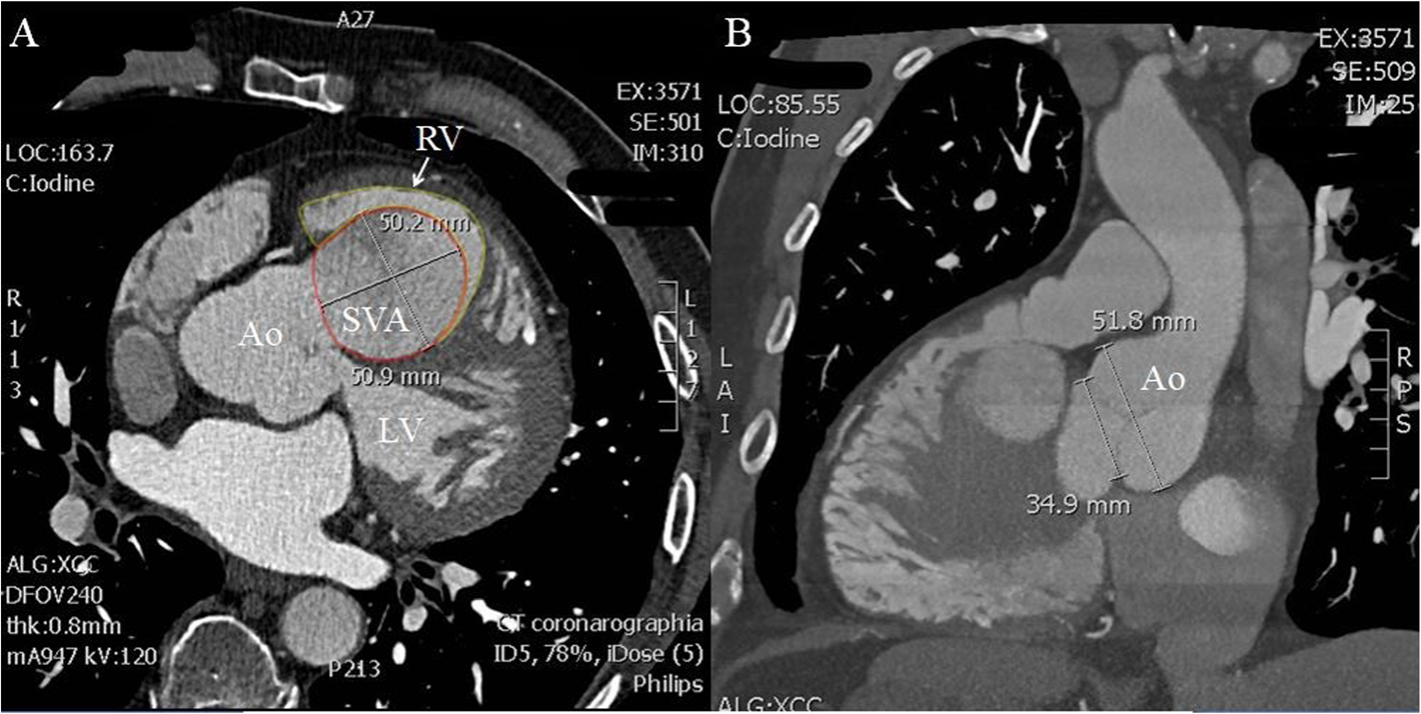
Computed tomography angiography. a – Transverse section showing the origin of the SVA from the right aortic cusp, occupying most of the right ventricle (yellow line) which is visible in this incidence as a thin zone around the aneurysm (red line). b – Sagittal section showing the diameters of the aortic valve and aortic root at the level of the sinuses of Valsalva. SVA = sinus of Valsalva aneurysm; Ao = aorta; LV = left ventricle; RV = right ventricle
